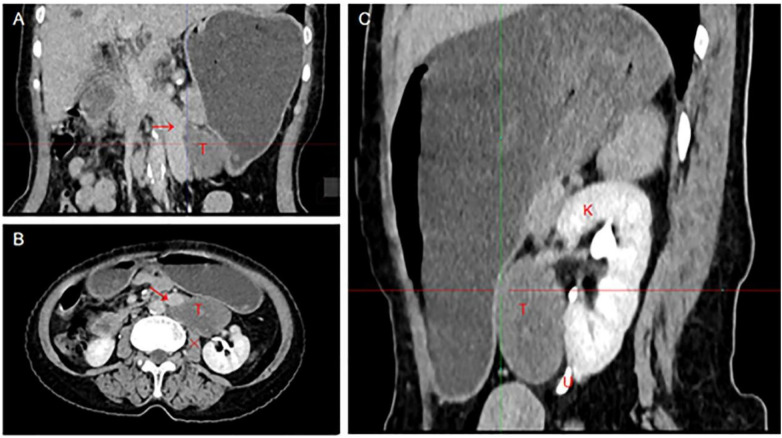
Enhanced CT image, (A) is coronal plane, (B) is transverse plane, (C) is sagittal plane. Arrow indicates IVC, T indicates tumor (CT attenuation values <40 HU), Fork indicates psoas muscle, K indicates kidney, and U indicates ureter.
